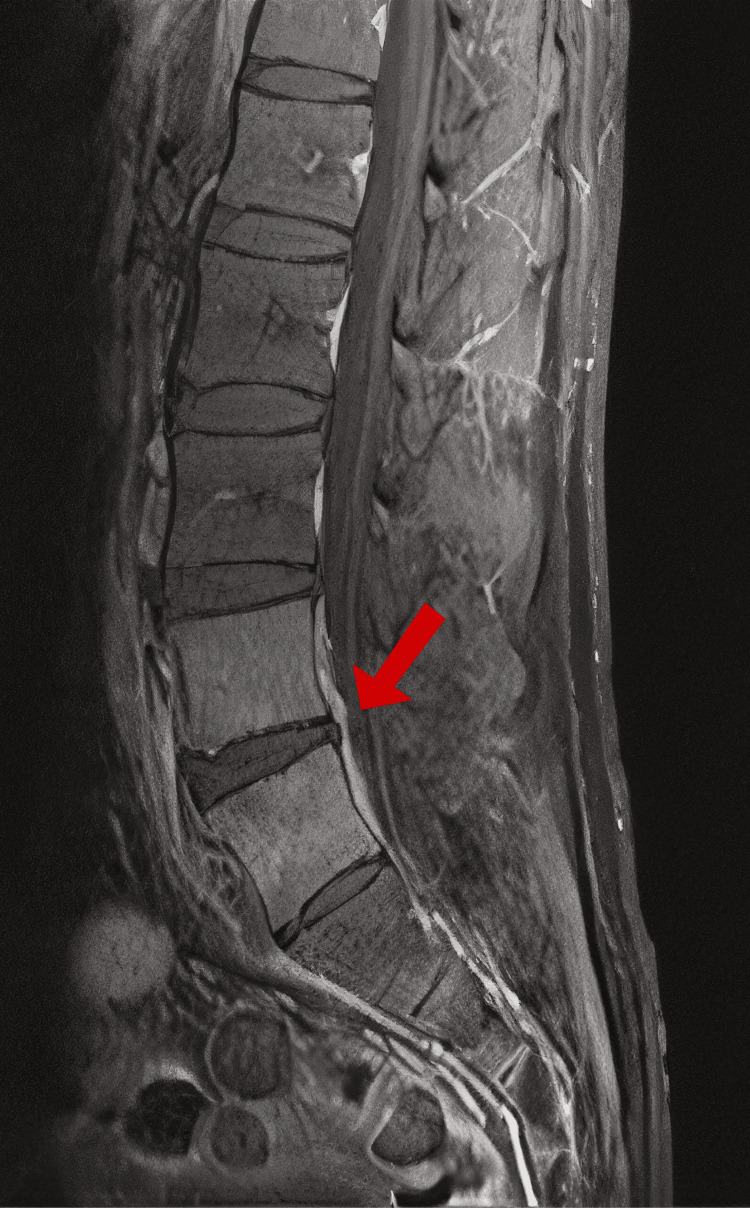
MRI lumbar spine, November 21, 2024 MRI: magnetic resonance imaging Sagittal T2-weighted MRI of the lumbar spine demonstrating hyperintense signal within the L4-L5 intervertebral disc and adjacent vertebral endplates with surrounding epidural soft-tissue thickening (arrow), findings consistent with vertebral discitis-osteomyelitis
